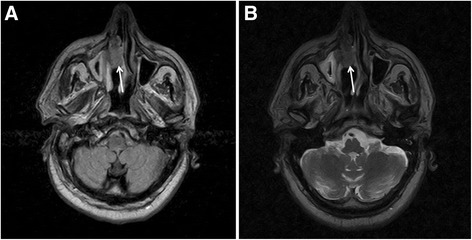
Magnetic resonance imaging with contrast of Case 2. Magnetic resonance imaging, axial view, with contrast medium showed hyperintense signal in T1 (a; *white arrow*) and fluid-attenuated inversion recovery sequences and hypointense signal in T2 (b; *white arrow*) in the right nasal fossa
